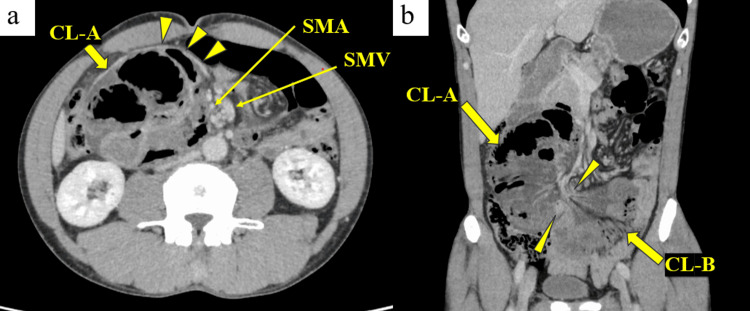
Contrast-Enhanced Computed Tomography (a) CT revealed dilated small bowel loops with diminished mural enhancement in the right upper abdomen, forming a CL obstruction (CL-A) with a sac-like appearance suggestive of encasement within a peritoneal sac (arrow). These loops displaced the SMV anteriorly from the dorsal aspect (arrowhead). The SMV was located to the left of the SMA (SMV rotation sign). (b) A second CL obstruction (CL-B), consisting of dilated loops with reduced mural enhancement, was identified in the left lower quadrant (arrow). The two narrowing points of CL-A and CL-B converged at a single location (arrowhead). CL, closed loop; SMA, superior mesenteric artery; SMV, superior mesenteric vein
